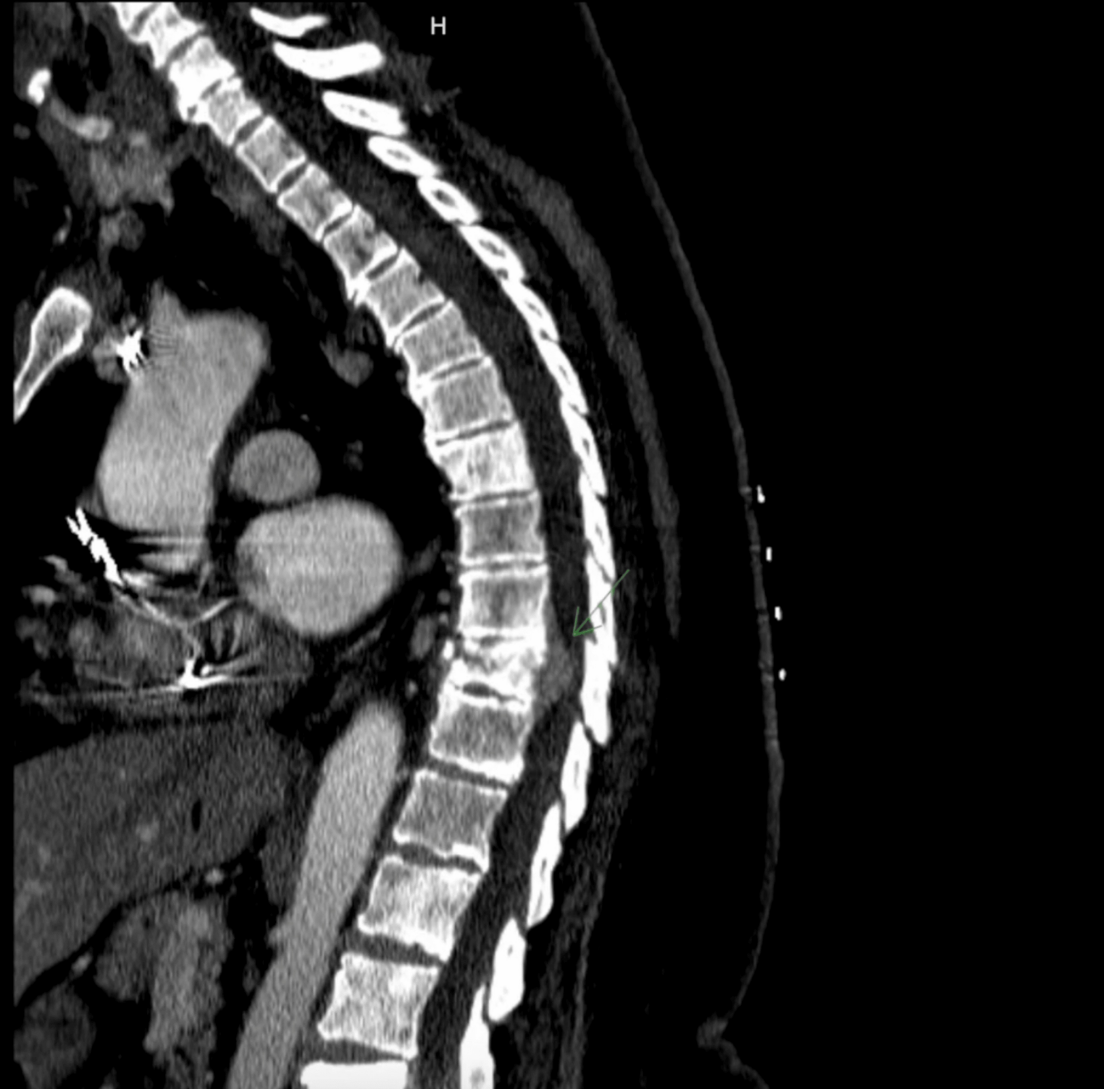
CT Scan Showing Intracanalicular Mass at T9-T11 With Fracture at T10
